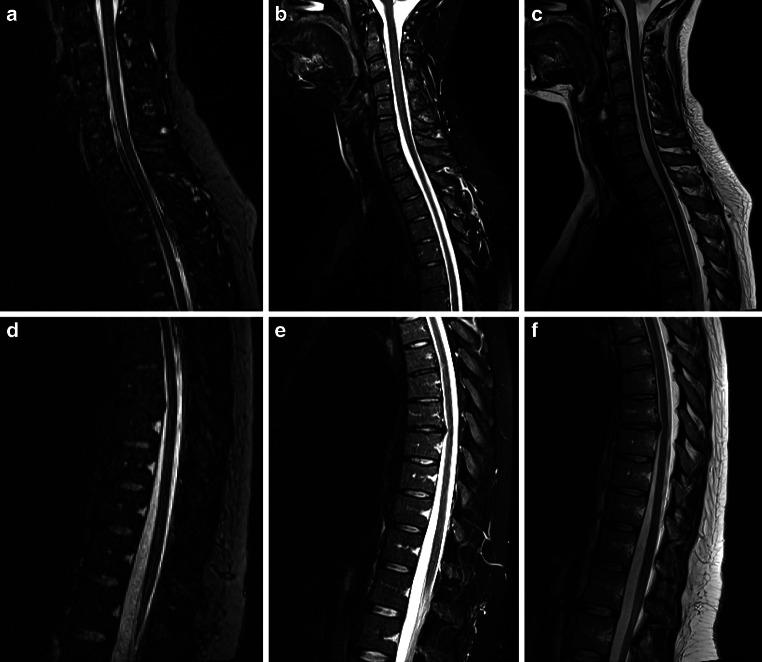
Example of a 30-year-old female patient with a 4-month history of multiple sclerosis (MS) but without spinal MS lesions. The expanded disability status scale (EDSS) was 1 and she had no disease-modifying treatment during the time of MRI. The imaging quality was rated good for the PSIR images (a and d) and for the STIR images (b and f). For the T_2_-w images (c and f) the imaging quality was rated good to very good
